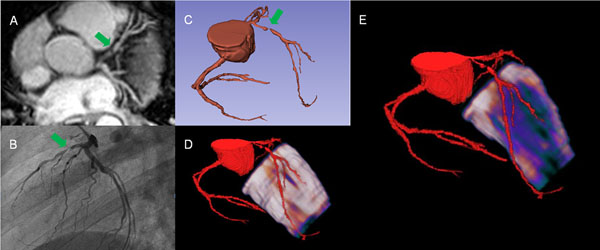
In this patient MRCA (A & C) and X-ray coronary angiography (B) reveal a high grade LAD stenosis (green arrows). 3D fusion of MRCA with CMR-perfusion shows no distinct perfusion deficit in the epicardial layer (D) but large subendocardial ischemia in the LAD territory (E).
